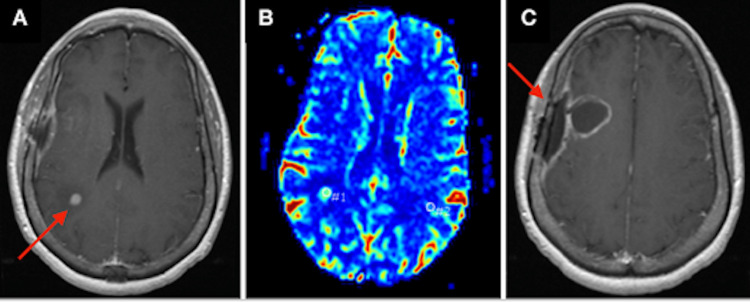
MRI brain showing interval development of an 8 mm rim-enhancing mass in the deep white matter of the right parietal lobe with extensive vasogenic edema. MR perfusion scan shows a significant increase of MR perfusion compared to normal brain, consistent with a new brain metastasis. (A) Axial view of the 8 mm rim-enhancing parietal mass (red arrow). (B) Axial view of MR perfusion scan showing increased perfusion of the mass. (C) Axial view demonstrating postoperative changes with a right frontal parietal craniotomy (red arrow).
